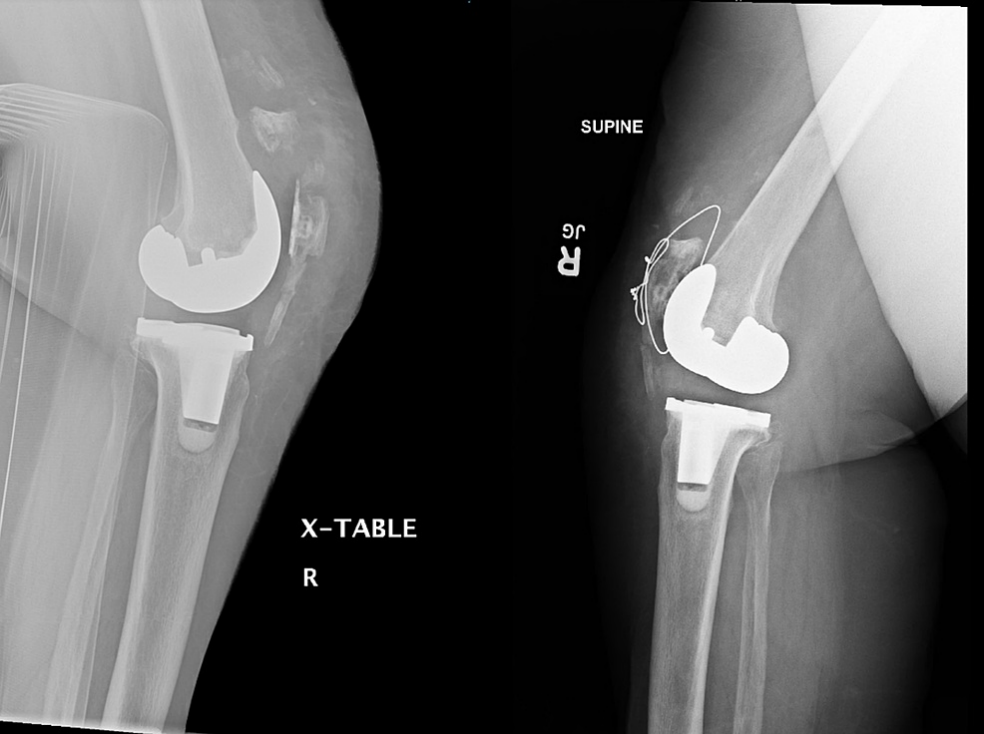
Readmission radiographs post primary total knee arthroplasty demonstrating a periprosthetic patella fracture (left); status post surgical fixation of periprosthetic patella fracture (right)
